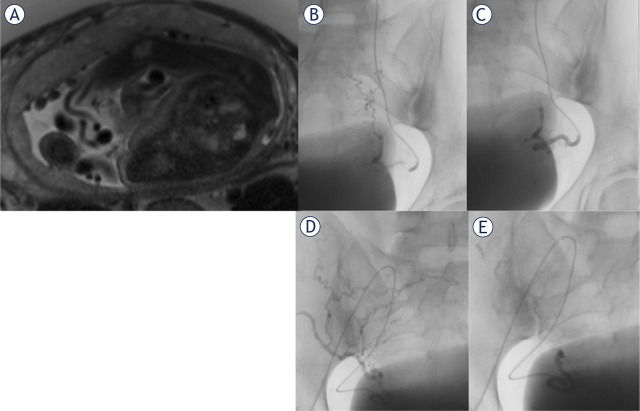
(A) Abdominal magnetic resonance imaging in a 35-year-old woman with singleton pregnancy at the 33rd week of gestation with suspected placenta accrete. The placenta covers the whole anterior part of the uterine wall. On T2-weighted MR images, there is marked thinning of the myometrium at the placental-myometrial interface, with MRI signs of placenta accrete and placenta increta. On the left cranial part of the junction, there is suspected placental invasion through the entire uterine wall, suspicious for placenta percreta. (B-E) Selective uterine angiography and embolization following delivery in the same 35-year-old woman with placenta accrete. Left (B) and right (D) uterine angiogram confirming the diagnosis of RPOC with numerous voluminous spiral arteries before prophylactic embolization. Left (C) and right (E) post-embolization angiogram after successful selective uterine artery embolization with absorbable gelatin sponge particles to reduce vascularity prior to surgical removal. After embolization, the placental tissue was successfully resected, and hysterectomy was prevented.
